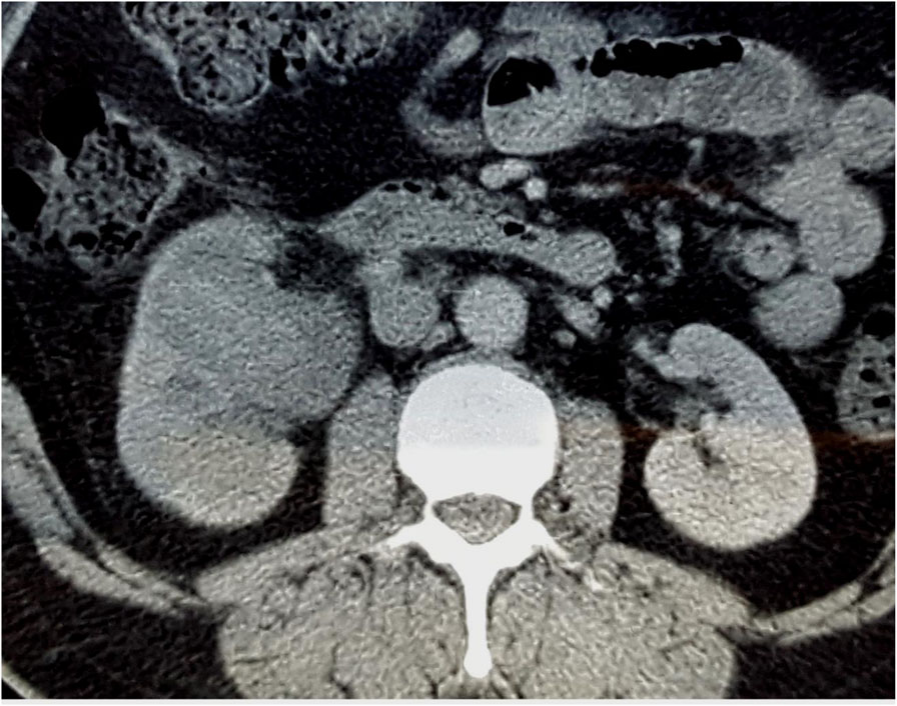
Contrast-enhanced computed tomography scan showing a slightly enhanced mass of the mid-portion of the kidney
